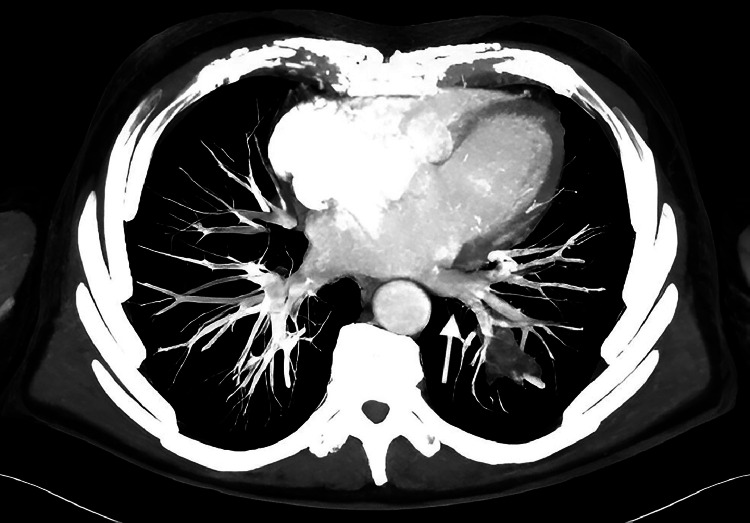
CT axial MIP demonstrated that lesion's venous drainage run from a pulmonary vein to left atrium (arrow).
